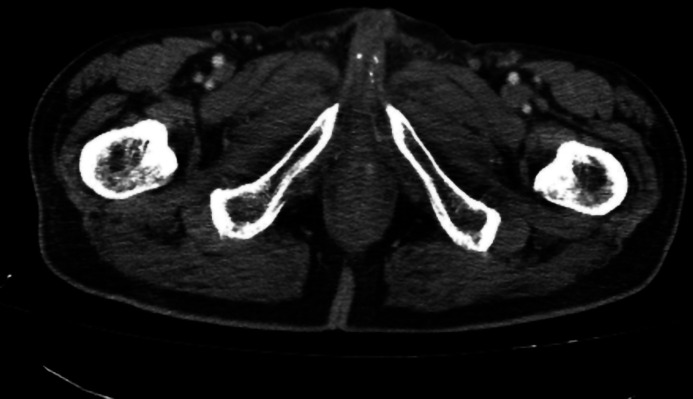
Computed tomography images show calcified Peyronie's plaques on both sides of the penis, multiple on the left-sided.
